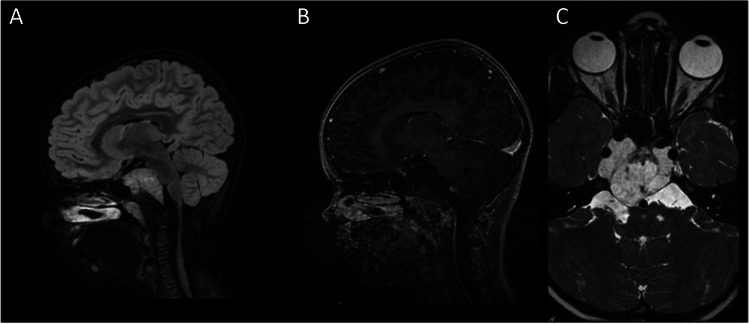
Preoperative MRI showing a clivus chordoma in A and B sagittal view and C axial view
